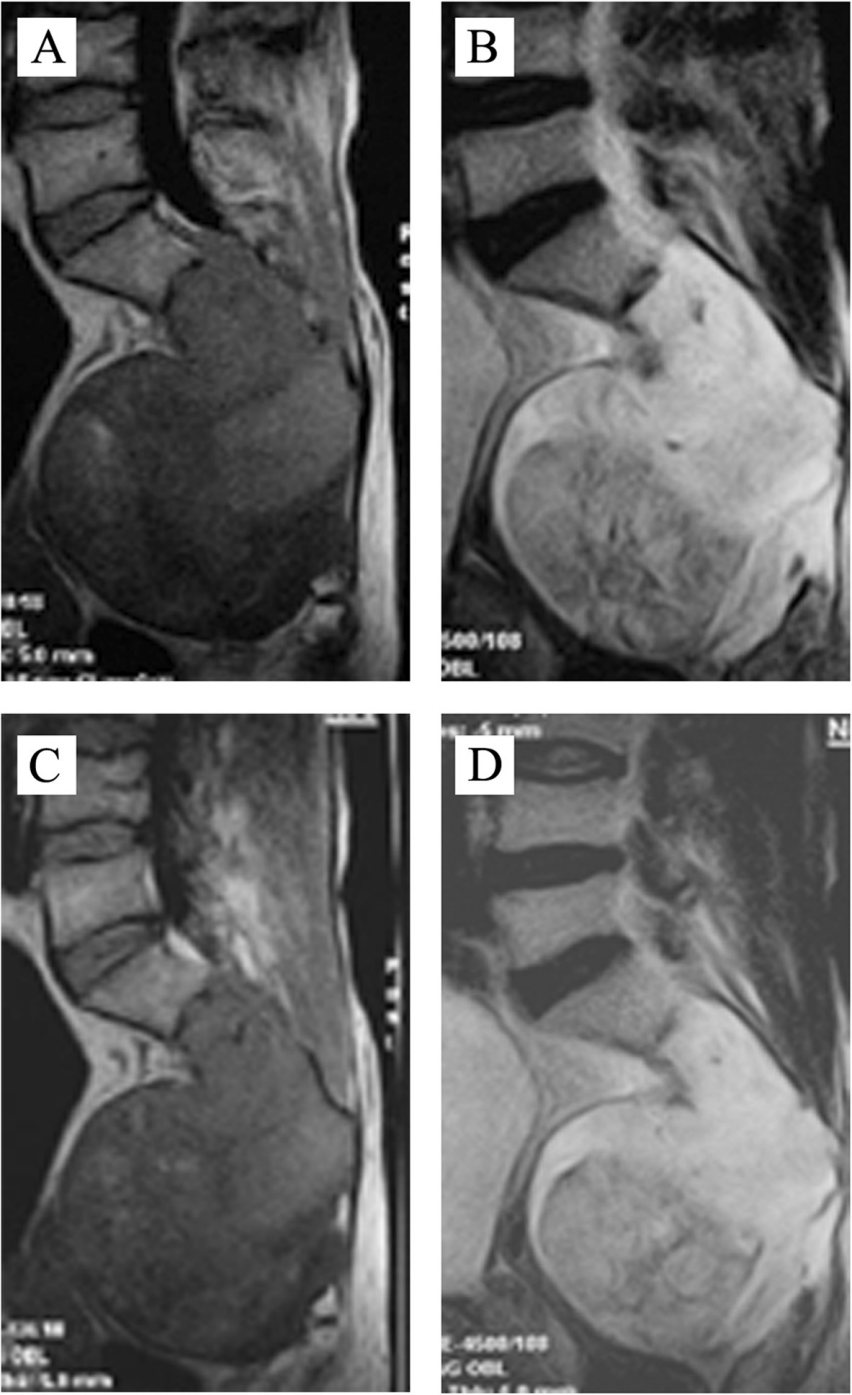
The computerized tomography (CT) scans of patient B before and after gelatin sponge particle (GSPs) implantation. Patient B received thrice of GSPs implantation in July 2011, April 2012, and December 2013, respectively. a, b The CT scans taken in February 2011; c, d: The CT scans taken in January 2014
